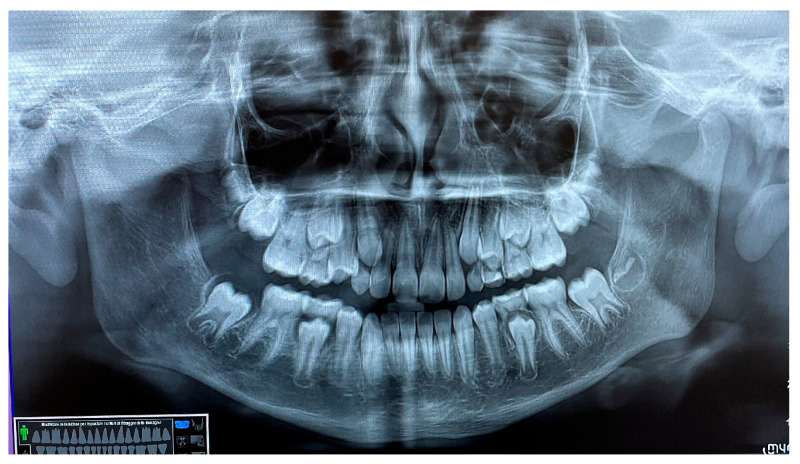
Orthopantomography X-ray after the treatment (clinical case #4).
